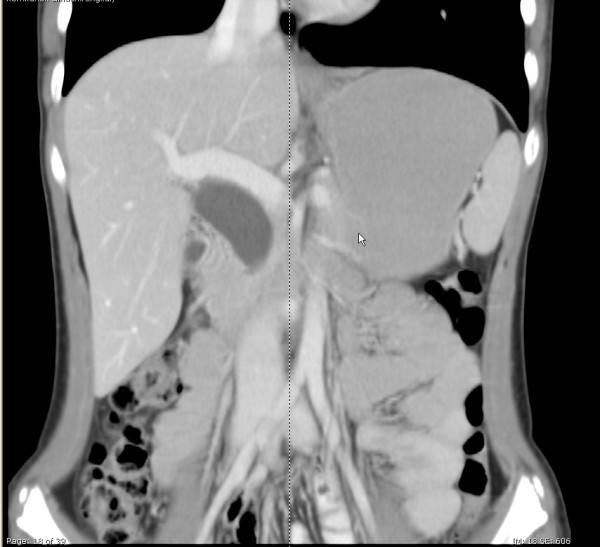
Computed tomography scan demonstrating the choledochal cyst type I.
